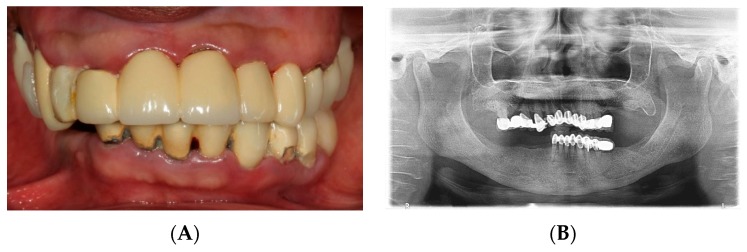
Initial examination. (A) Clinical presentation. (B) Panoramic radiograph.
